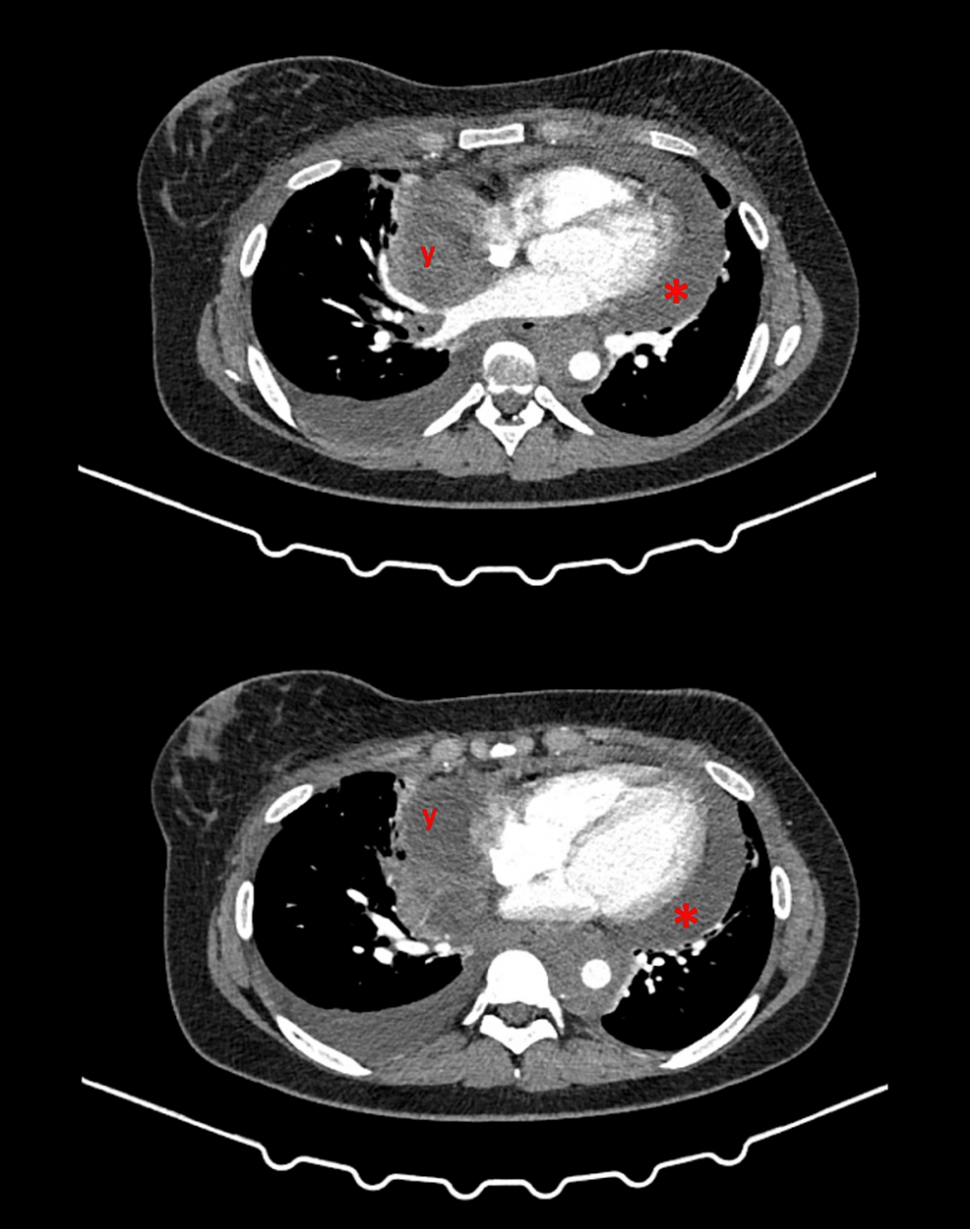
CT scan showing pericardial effusion (*) and a large mediastinal mass (y).
